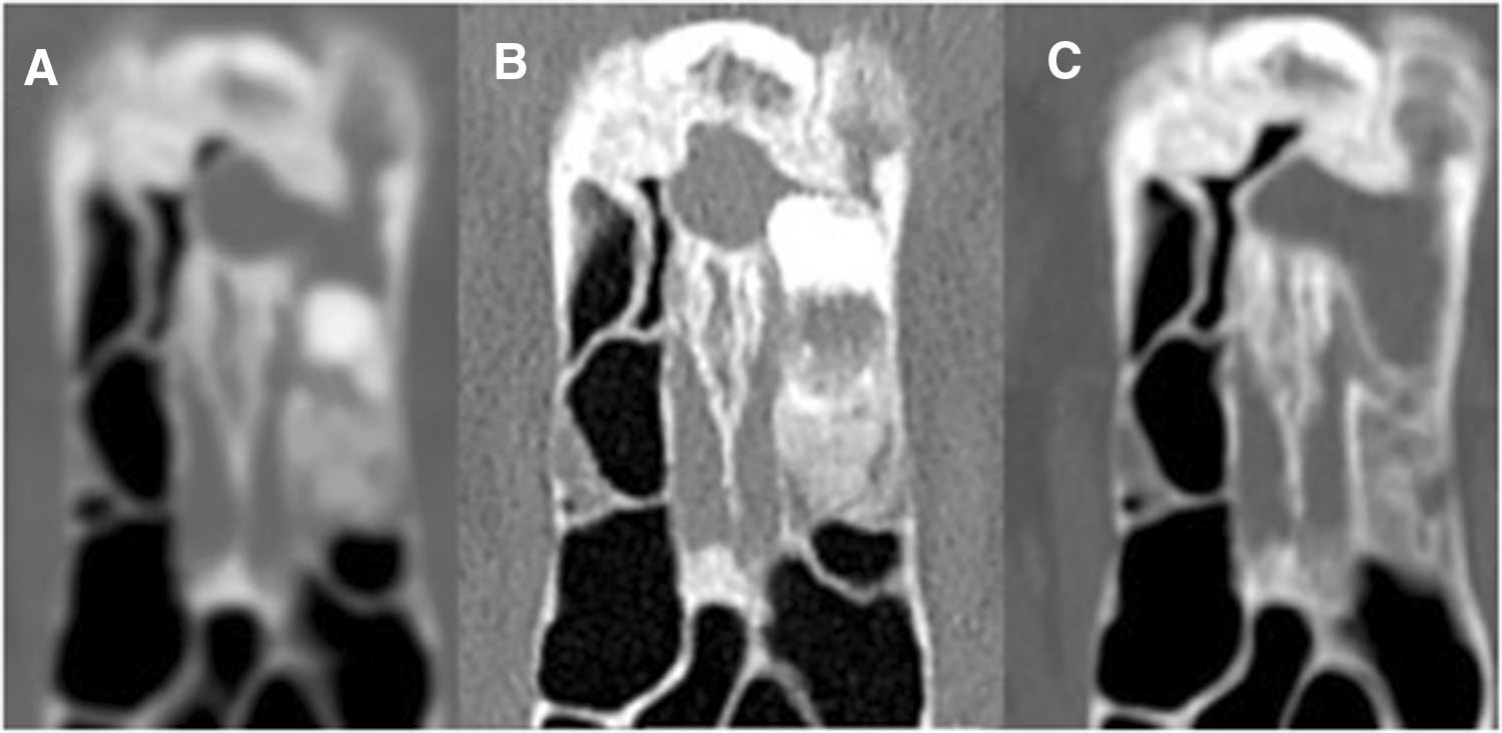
a, b Consecutive CT scans showing osteoma growth. c Postoperative scan showing opacification of the frontal sinus outflow tract
